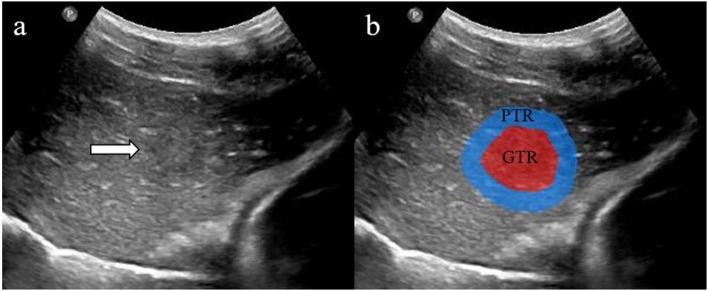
Two regions of interest (ROIs) were defined in grayscale ultrasound images (a). The red area shows gross-tumor region (GTR) signatures, and the blue area shows peri-tumoral region (PTR) signatures (b).
